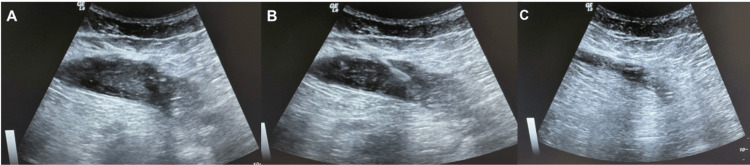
Ultrasound evaluation of the abdominal wall abscess. (A-B) Transverse greyscale images demonstrate a heterogeneous, hypoechoic, loculated collection within the left lower abdominal wall, suggestive of an organized abscess. (C) Post-drainage view showing the catheter tract and partial resolution of the collection with decreased fluid content.
